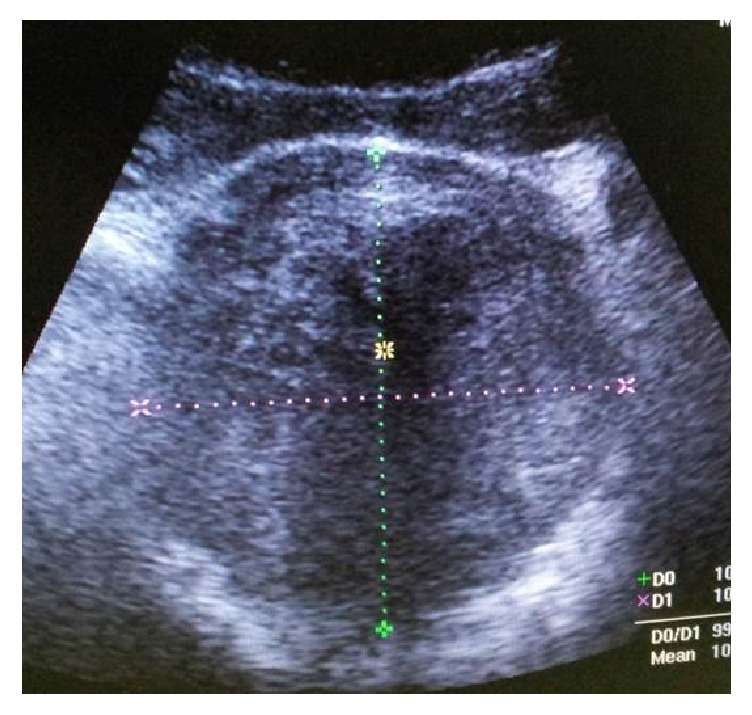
Abdominopelvic ultrasound study showing a well-defined uterine mass measuring 10,5 cm × 10,6 cm.
